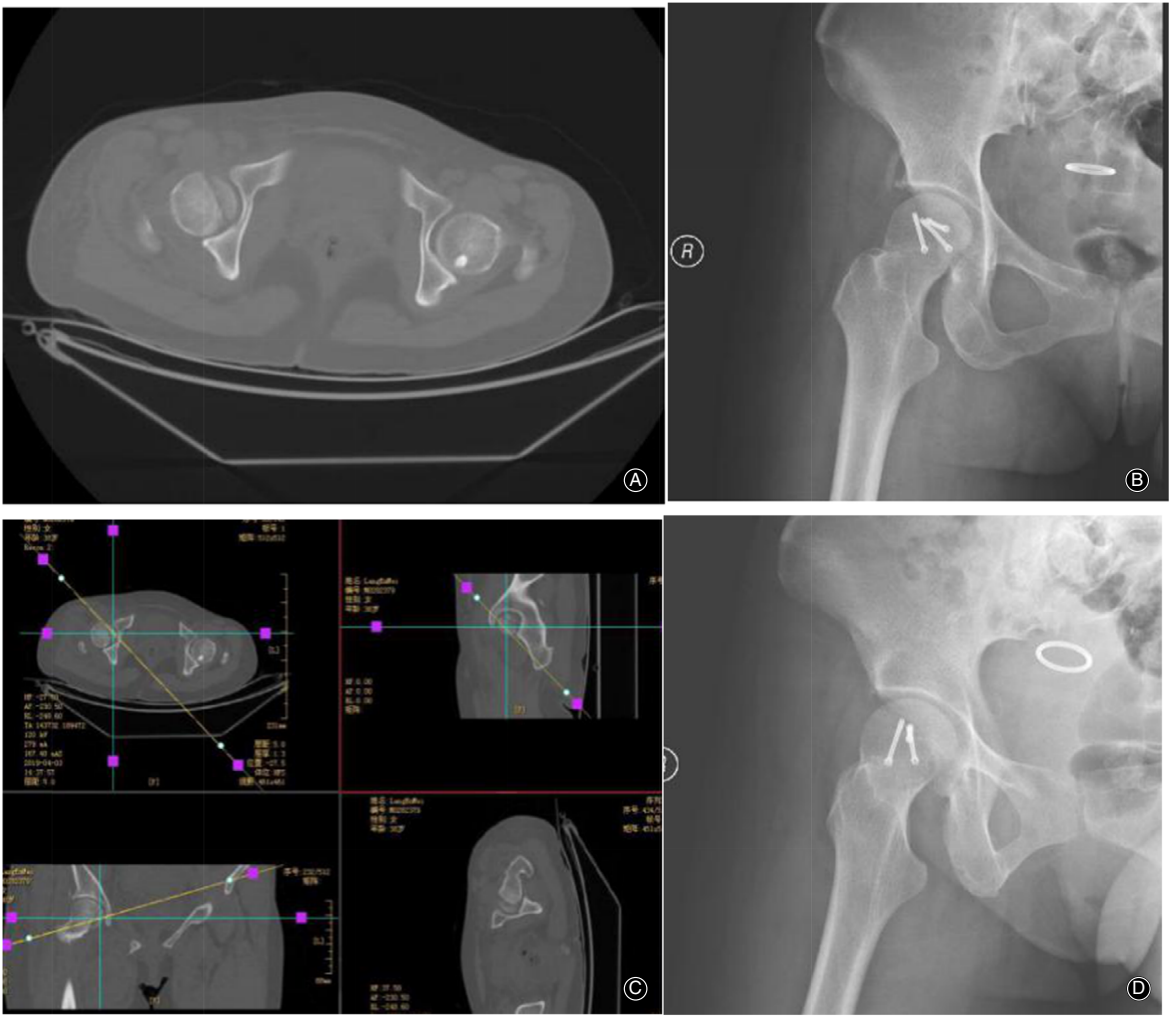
Femoral head fracture Pipkin II type imaging data: (A, B) preoperative CT and reconstruction; and (C, D) anterior and lateral X‐ray of the hip after operation.
